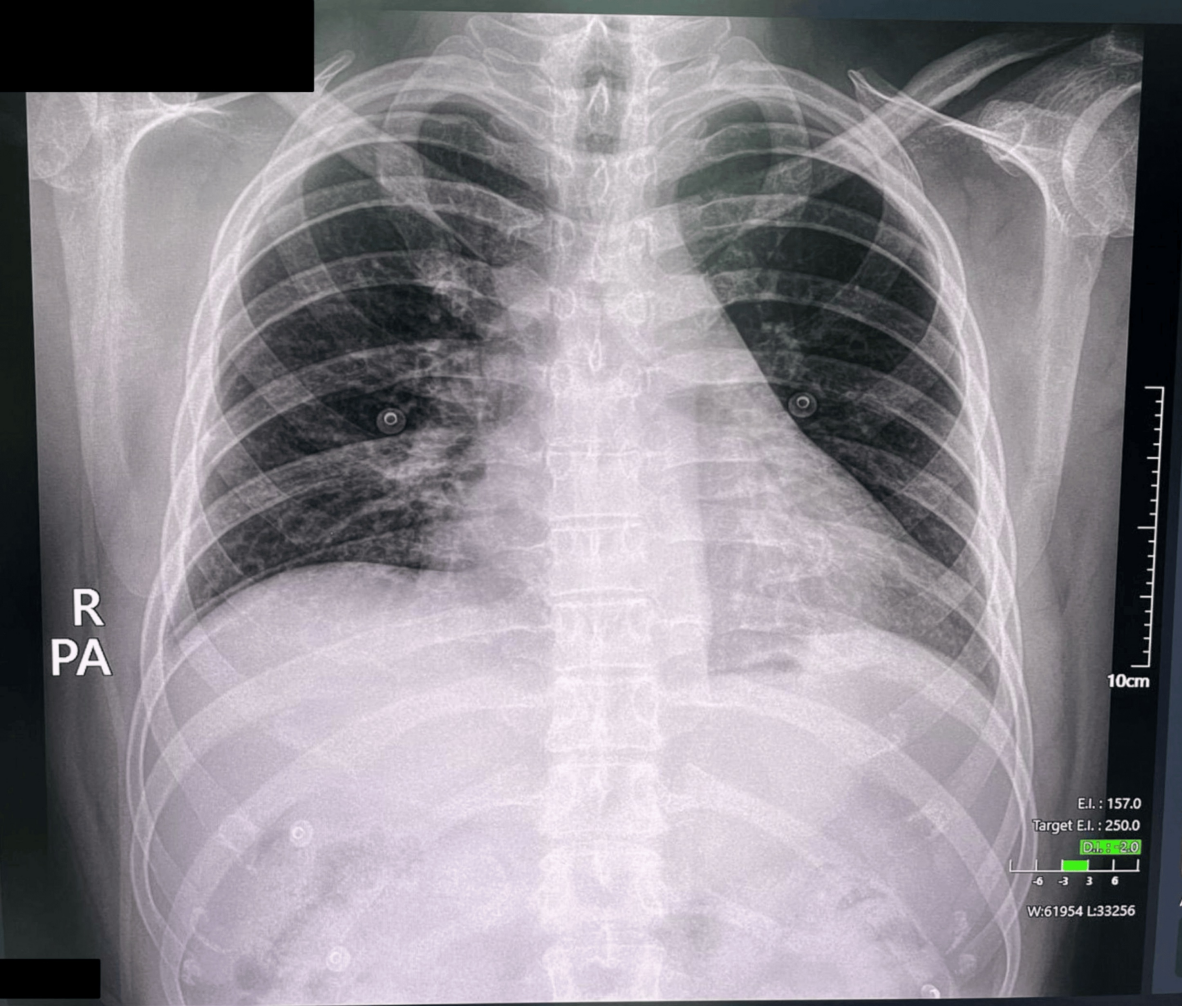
Follow-up chest X-ray. PA: posteroanterior; R: right.
